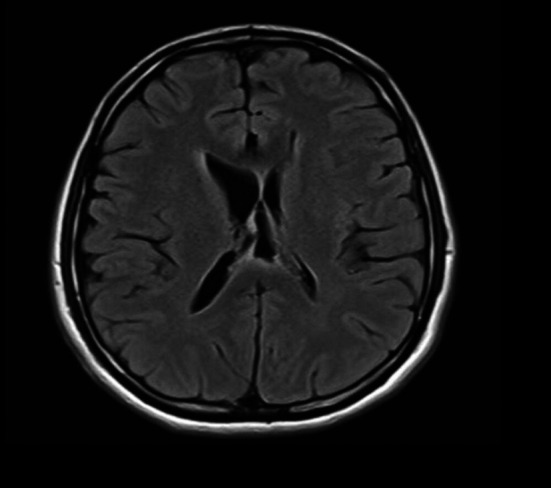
MRI brain scan axial T1 image demonstrates resolved hydrocephalus at 6 months follow up post APC resection.
